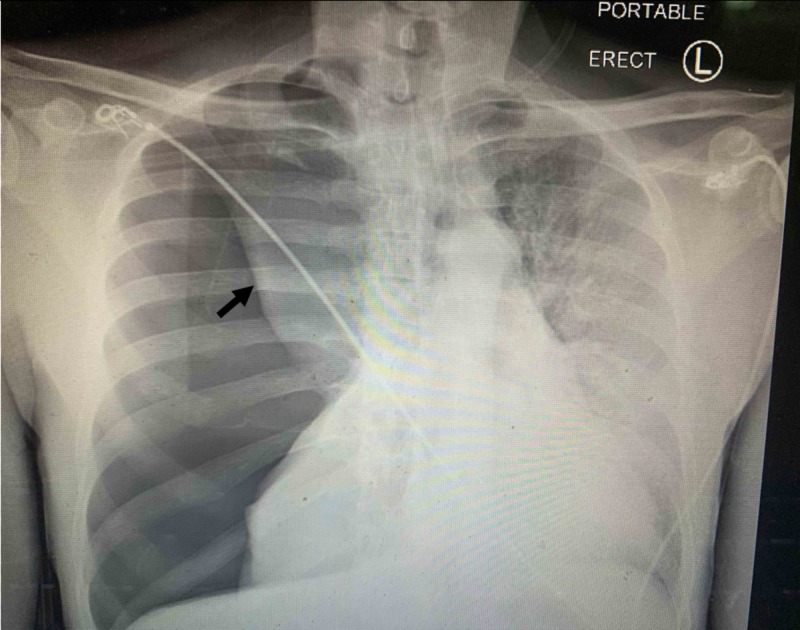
Chest X-ray showed massive right-sided tension pneumothorax with collapse of the right lung (arrow) and leftward shift of the mediastinum
